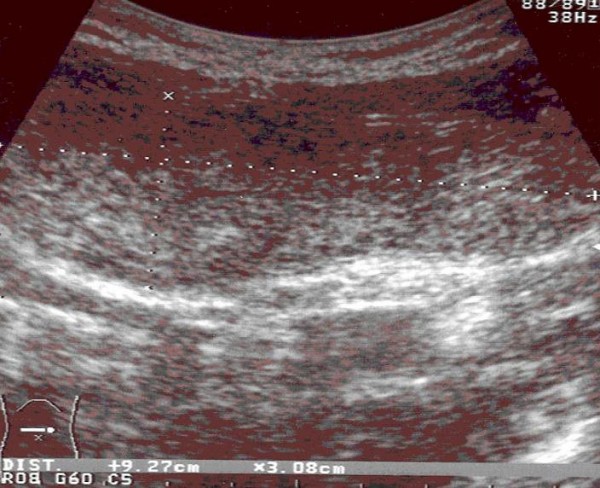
Longitudinal ultrasonogram of the abdomen showing continuity of the stomach and its contents in the periumbilical region.
